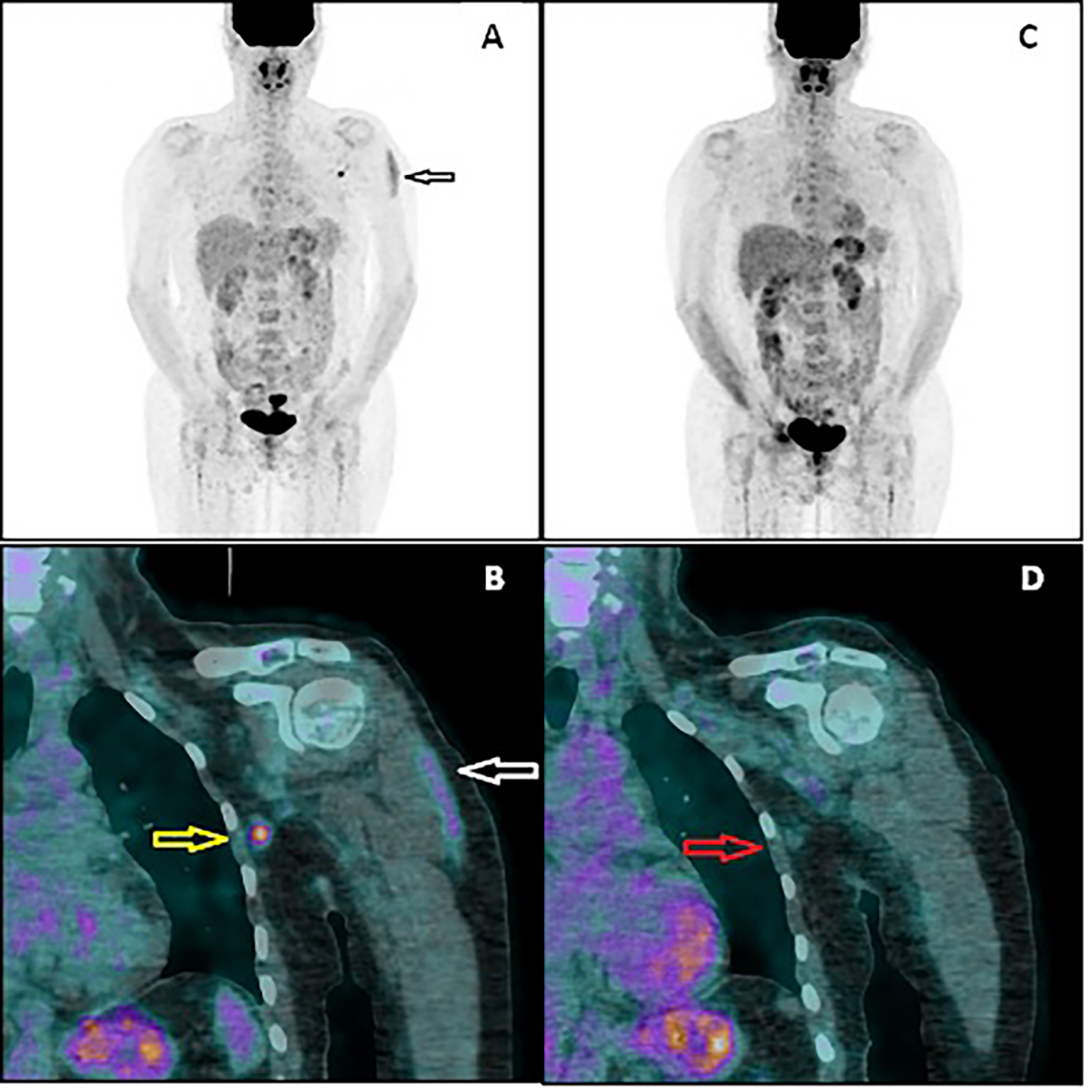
PET/CT image performed 4 days after vaccination (A, B). The multiple-intensity projection image (A) showing increased ^18^F-FDG uptake in the left deltoid muscle (black arrow) and in the left axillary region. Fused coronal image (B) showing the uptake in the muscle (white arrow) and in the axillary lymph node (yellow arrow). PET/CT image performed 32 days after vaccination (C, D). Both the muscular uptake and nodal uptake have disappeared. The referred axillary lymph node (red arrow) shows similar morphology but no ^18^F-FDG accumulation.
